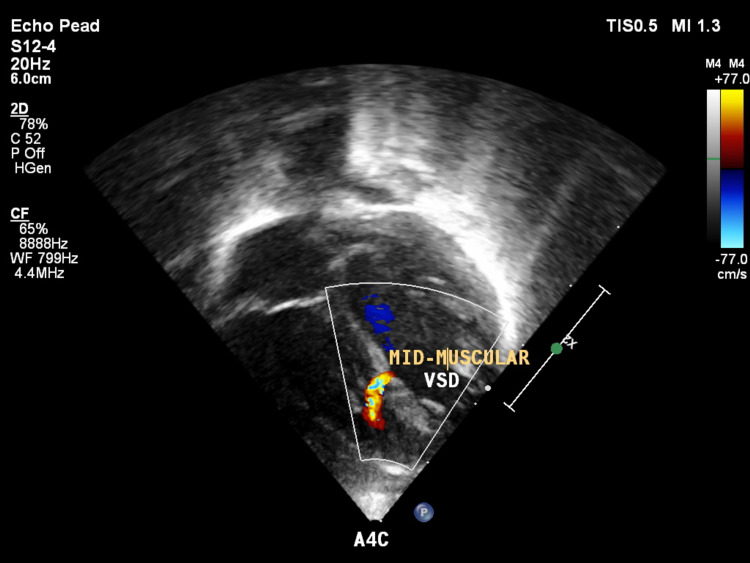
Two-dimensional echocardiogram image showing the mid-muscular ventricular septal defect.
